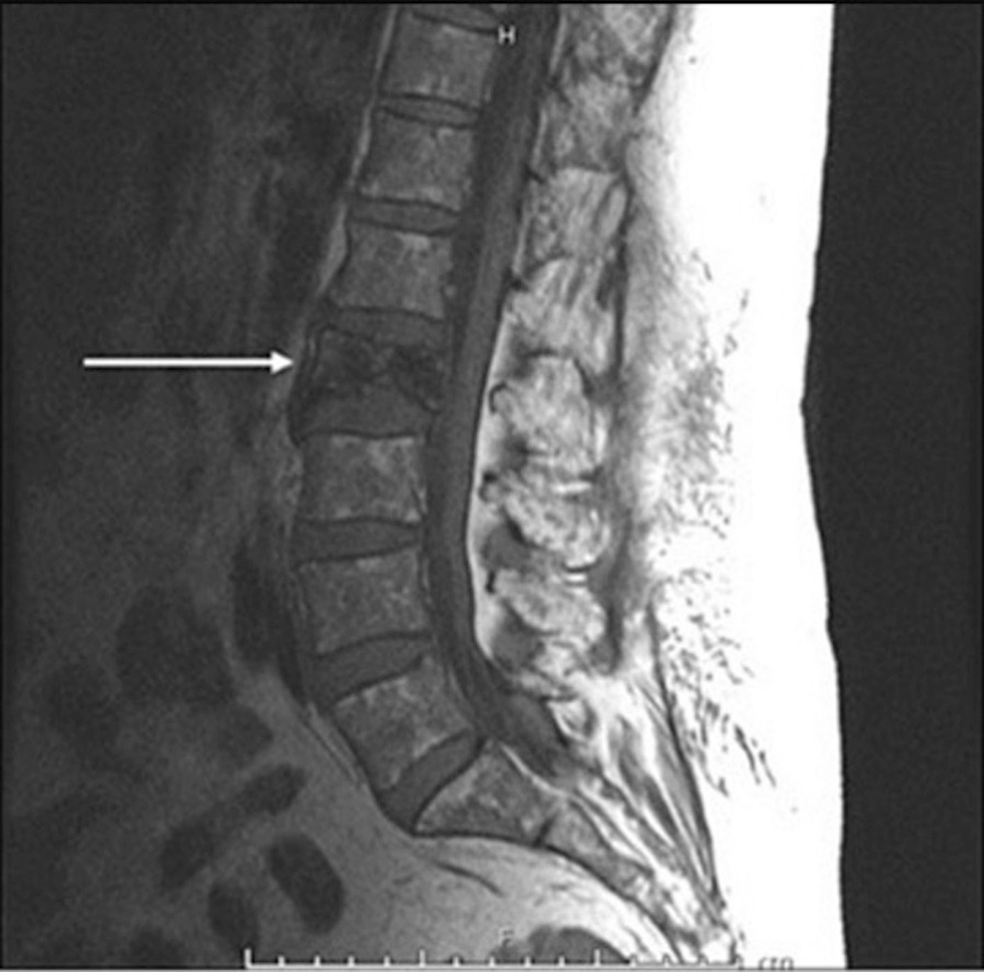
MRI of the lumbar spine with contrast showing L2 increased heterogeneity (white arrow), suggestive of metastasis. MRI: magnetic resonance imaging
